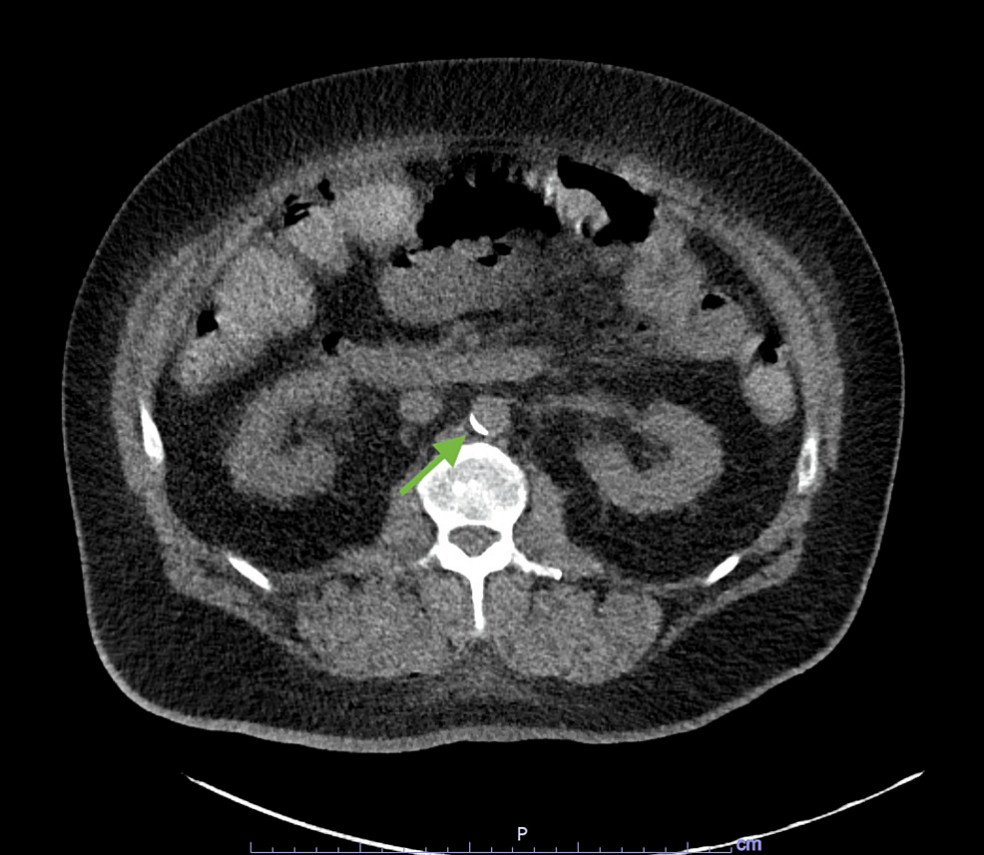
CT angiogram of the abdomen. Short segment occlusion of the origin of the celiac artery with distal reconstitution and associated periceliac infiltration.
